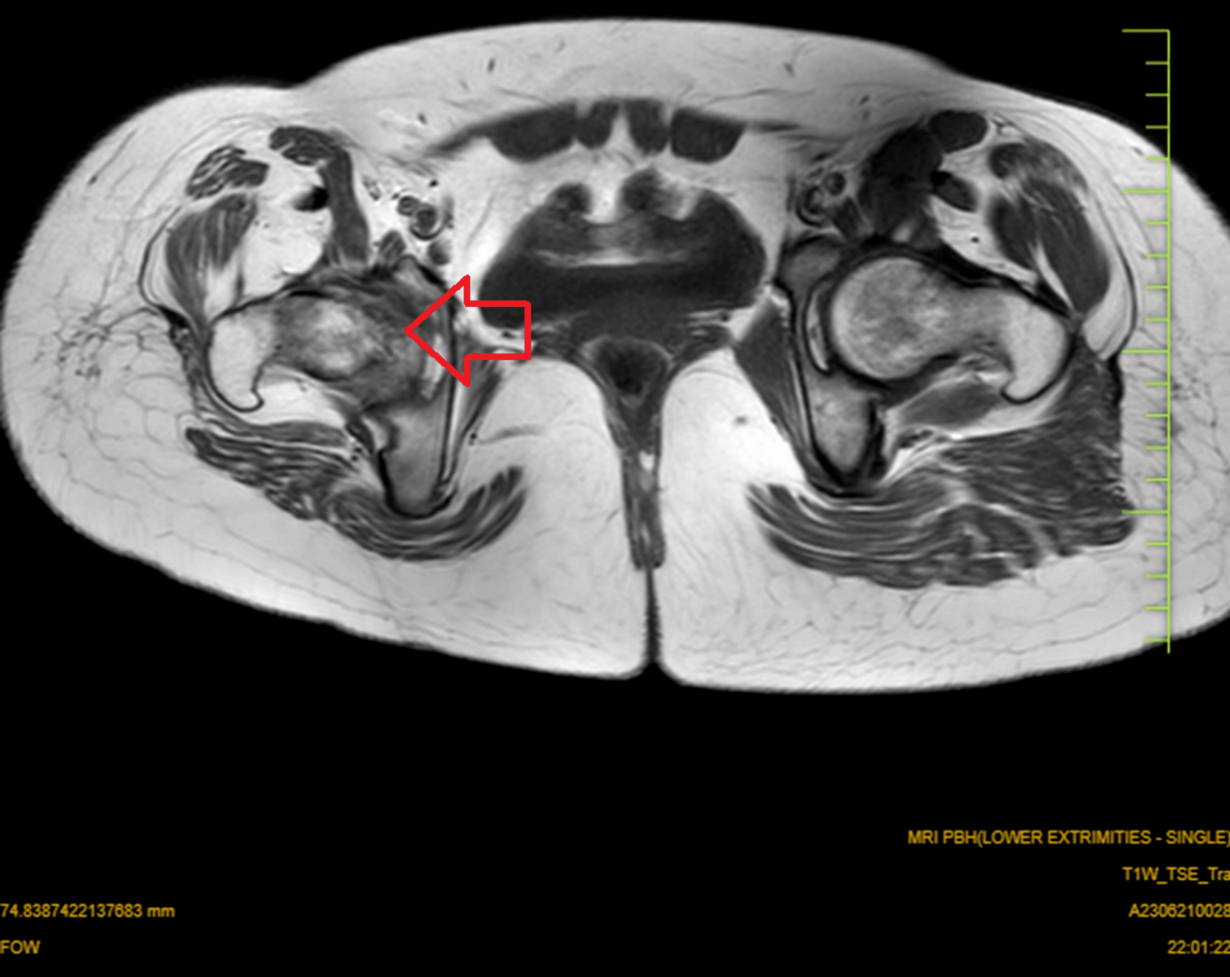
MRI of the pelvis with both hip axial sections shows reduced joint space on the right side as compared to the left side. MRI: magnetic resonance imaging
